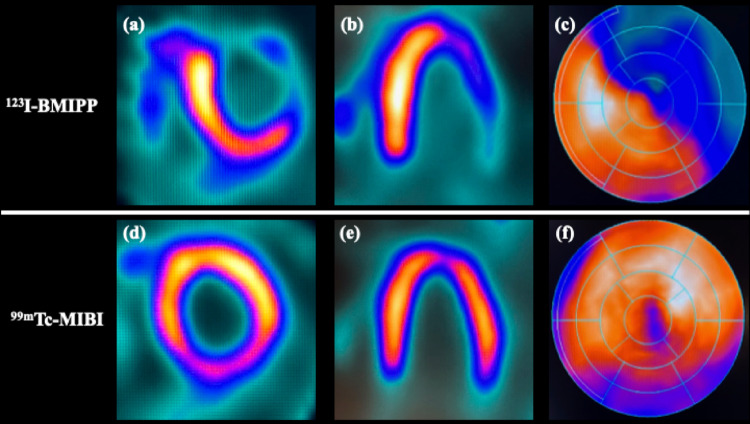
Dual myocardial scintigraphy demonstrating metabolic–perfusion mismatch. (a) ¹²³I-β-methyl-*p*-iodophenyl-pentadecanoic acid (BMIPP) single-photon emission computed tomography (SPECT) in the axial view demonstrates a well-demarcated reduction in tracer uptake in the anterior to lateral wall of the left ventricle. (b) ¹²³I-BMIPP SPECT in the coronal view shows concordant reduction in tracer uptake in the anterior to lateral left ventricular wall. (c) ¹²³I-BMIPP SPECT polar map (bull’s-eye plot) demonstrates a regional reduction in tracer uptake in the corresponding anterior to lateral left ventricular segments. (d) Corresponding axial ⁹⁹ᵐTc-methoxyisobutylisonitrile (MIBI) SPECT demonstrates preserved myocardial perfusion in the same region. (e) Corresponding coronal ⁹⁹ᵐTc-MIBI SPECT demonstrates preserved perfusion in the corresponding region. (f) ⁹⁹ᵐTc-MIBI SPECT polar map (bull’s-eye plot) demonstrates preserved perfusion in the same segments, facilitating objective visualization of the metabolic–perfusion mismatch and its regional extent. Note: As computed tomography-based attenuation correction was not available, mild inferior–posterior reduction on ⁹⁹ᵐTc-MIBI may reflect attenuation
